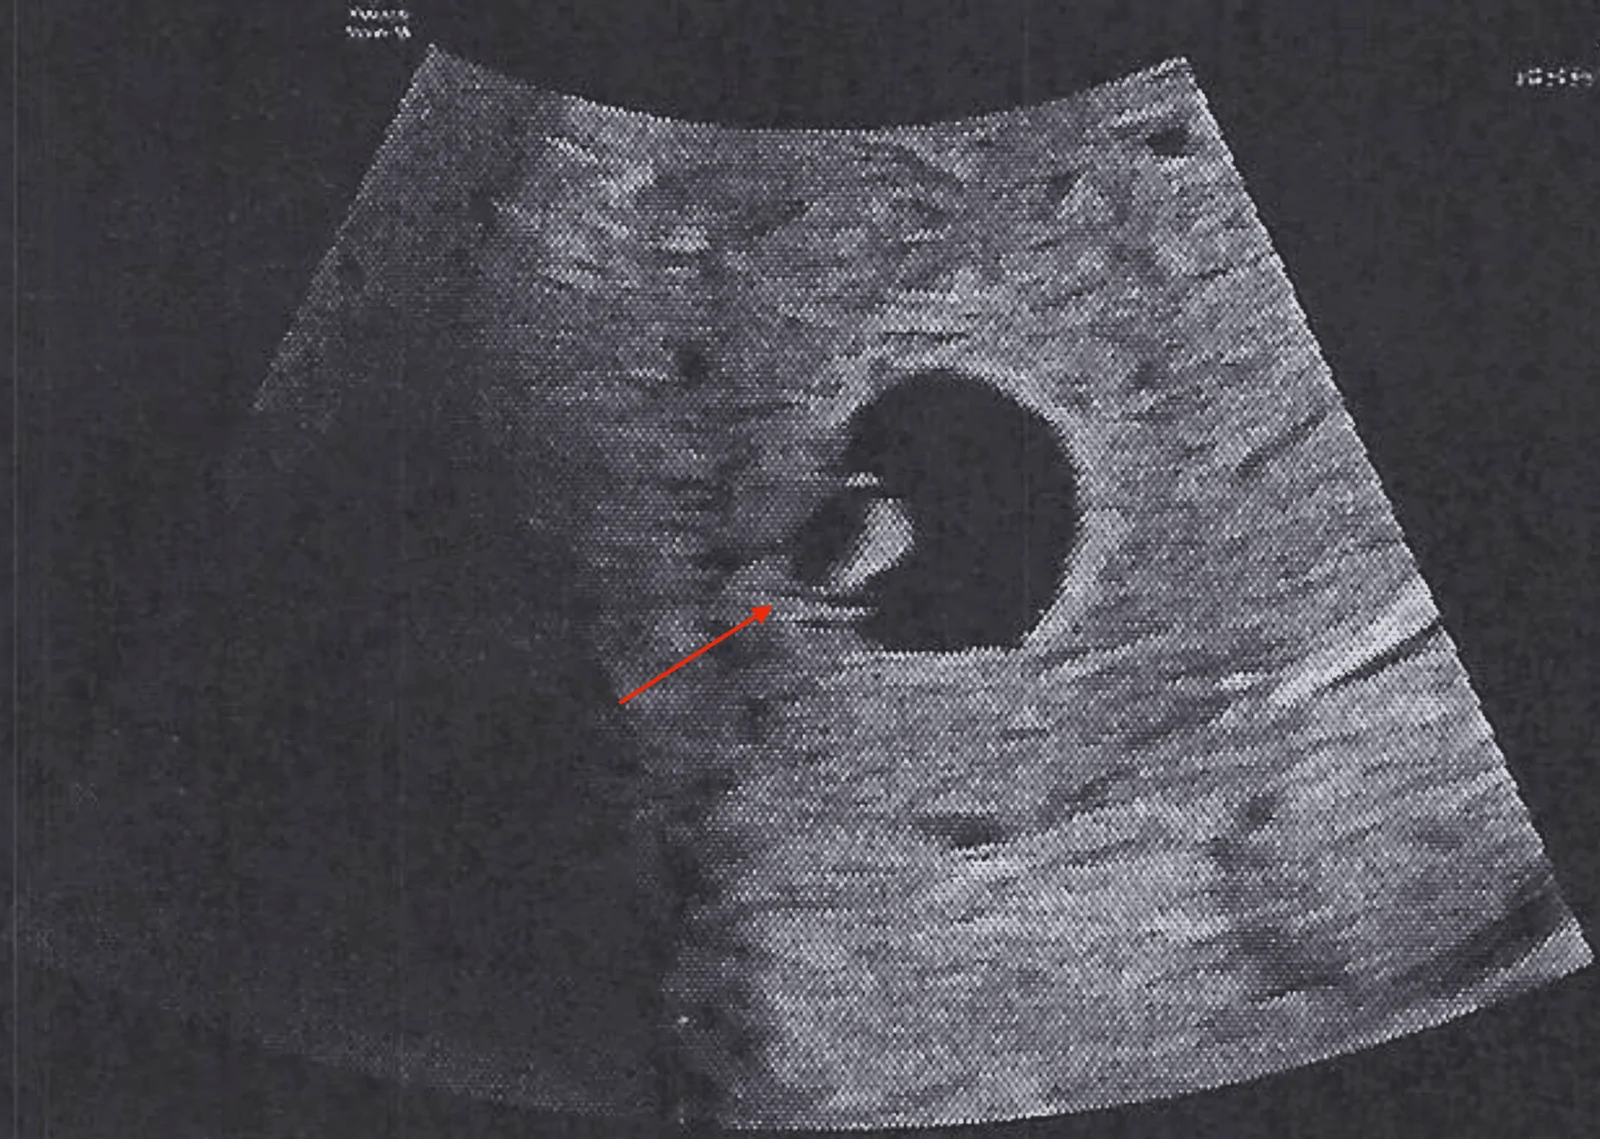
Obstetric ultrasound Obstetric ultrasound with a red arrow showing a gestation near the lateral edge of the uterus.
